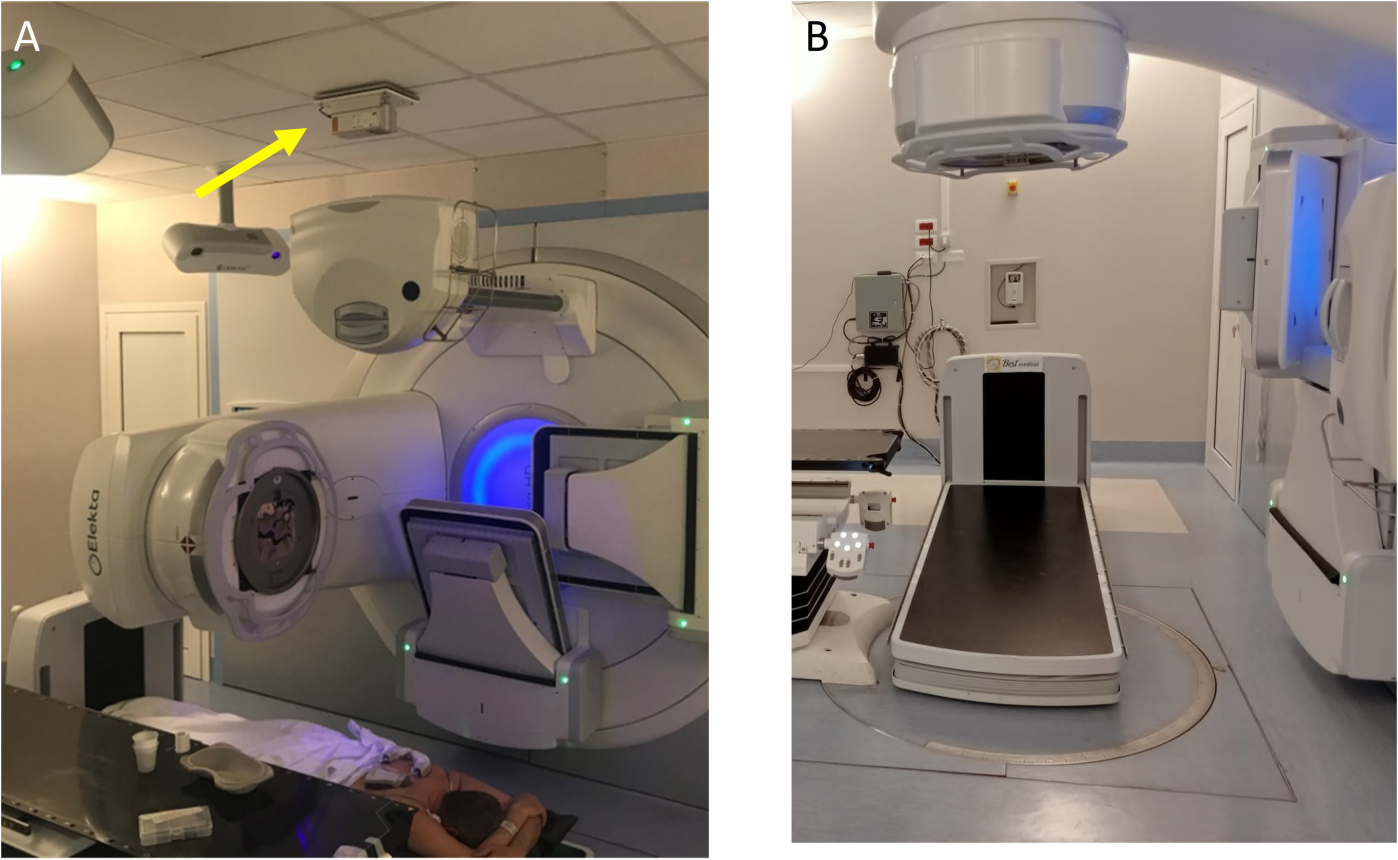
(A) Pt#1 in prone position on the dedicated couch coupled with a VERSA HD Linac (Elekta, Stockholm, Sweden). A dedicated additional laser, visible on the bunker’s ceiling and indicated with yellow arrow in panel (A), enables the patient alignment when placed on the TBI couch. With the gantry rotated 270°, the cone beam-CT tube allows the delivery of a kV X-rays beam for the setup imaging. At the end of this procedure, the cone beam-CT tube is retracted, and the gantry is rotated 0° for the TBI delivery in the prone position. (B) Positioning of dedicated TBI couch for patient treatment with Elekta couch retracted.
